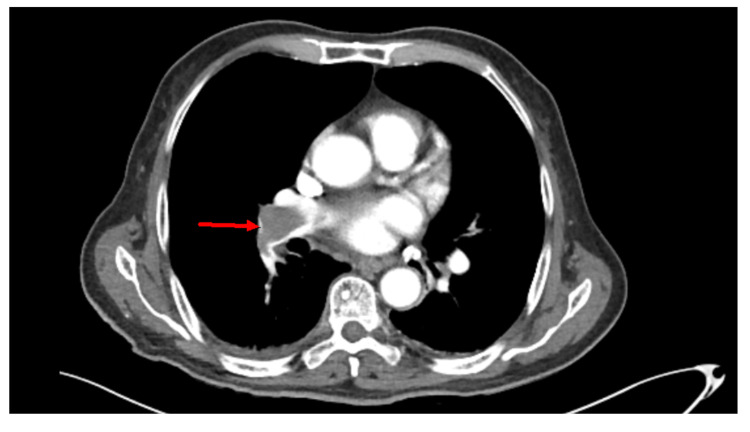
Incidental finding of a saddle embolism on an abdominal and pelvis CT scan
